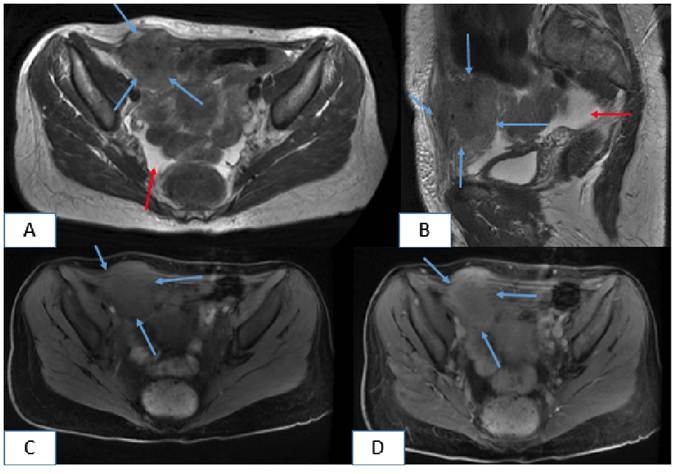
Axial (A) and sagittal (B) T2-weighted MRI reveals a hyperintense abdominal wall mass (blue arrows) extending into the adjacent intra-abdominal and subcutaneous regions, with associated pelvic fluid accumulation (red arrows). Axial precontrast (C) and postcontrast (D) T1-weighted MRI shows the enhancement of the mass.
